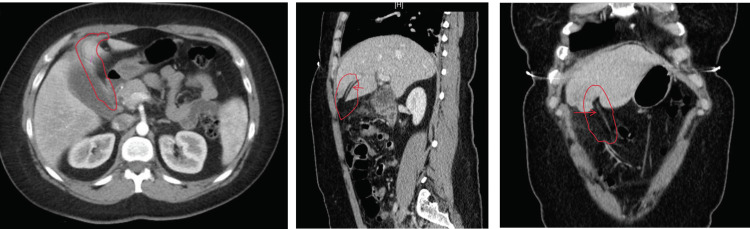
Computed tomography (CT) scans demonstrating the hepatic falciform artery (arrow)
